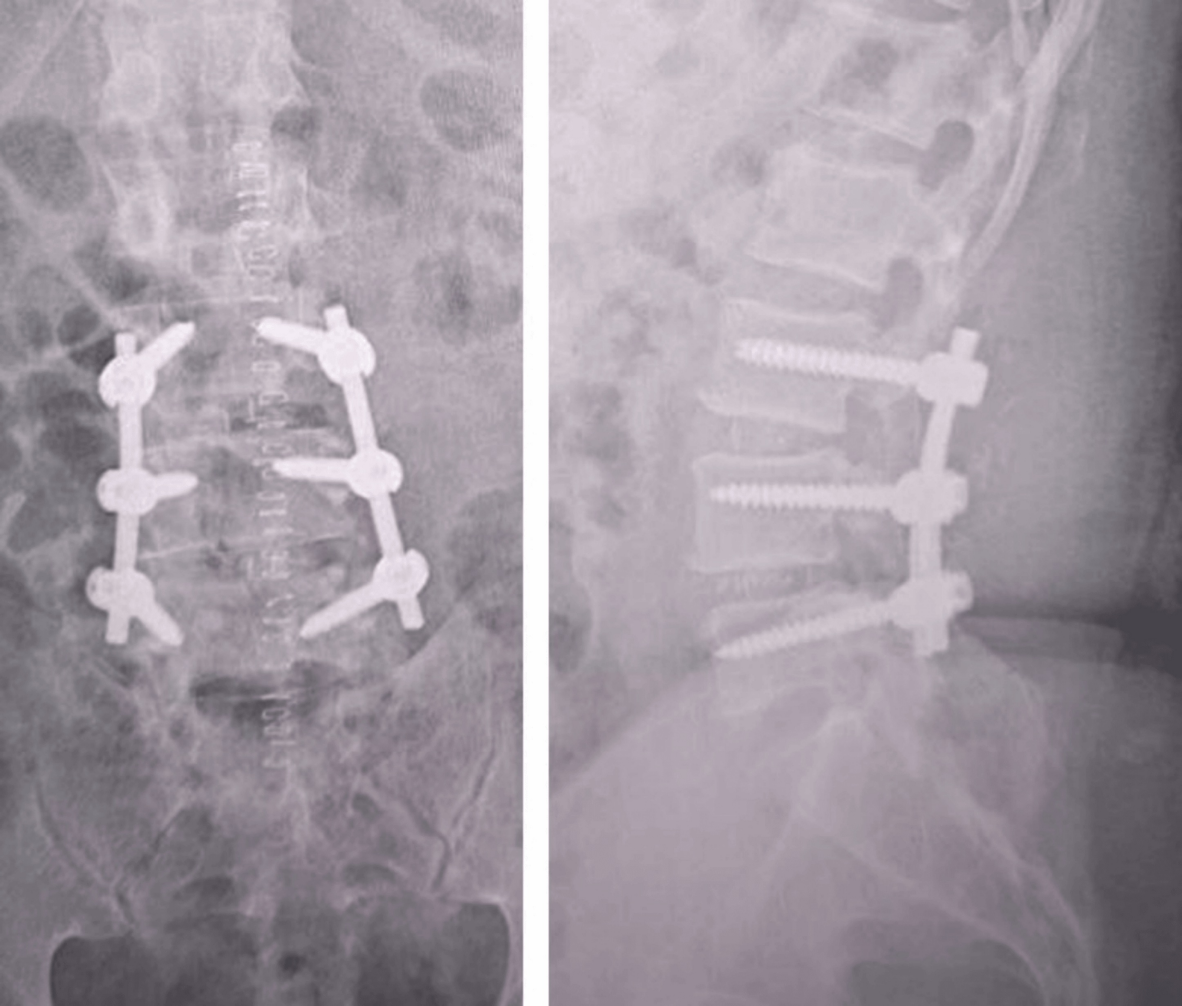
Postoperative X-ray of the lumbar spine
